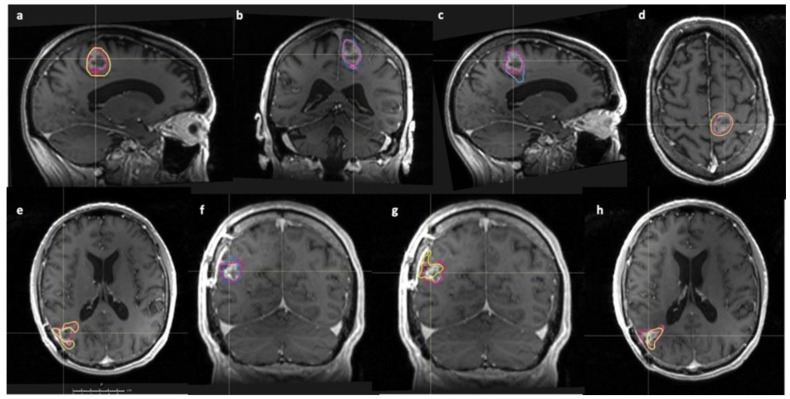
Selected Gd-T1 sagittal, axial and coronal images taken at different study timepoints, with PET or DCE-MRI volumes overlaid for GBM 1 (top row) and GBM 5 (bottom row). Yellow—PET (3 × SUV_max_) volume; Pink/red—Gd-T1 volume; Blue—K^trans^ volume. Top row—GBM 1; (a) –pre-RT sagittal, (b)—coronal pre-RT, (c)—sagittal mid-RT and (d)—axial mid-RT images. Bottom row—GBM 5; (e)—axial pre-RT, (f)—coronal mid-RT, (g)—coronal mid-RT and (h)—axial post-RT images.
